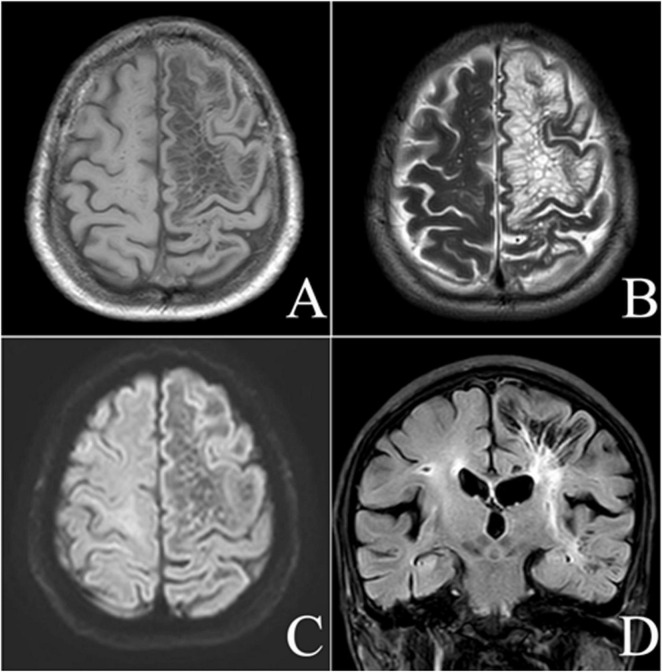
Male, 72-year-old, discomfort and a scalp mass for more than 4 months. MRI axial T1-weighted (A), axial T2-weighted (B), diffusion-weighted (C), and coronal fluid-attenuated inversion recovery-weighted (D) images showing a giant tumefactive perivascular space (GTPVS) located in the left cerebral hemisphere, which shows isointense relative to cerebrospinal fluid (CSF). White matter high signal can be seen surround the lesion. Cavity foci are identified in the right radiocoronal area and adjacent to the lesion.
